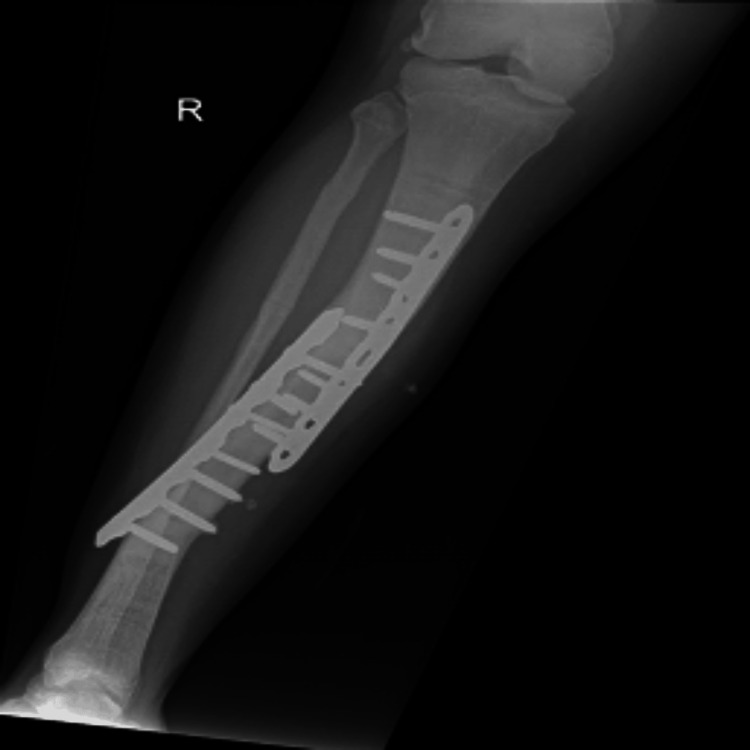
Radiographic Evidence of Surgical Fixation in Osteopetrosis-Related Fracture Management
